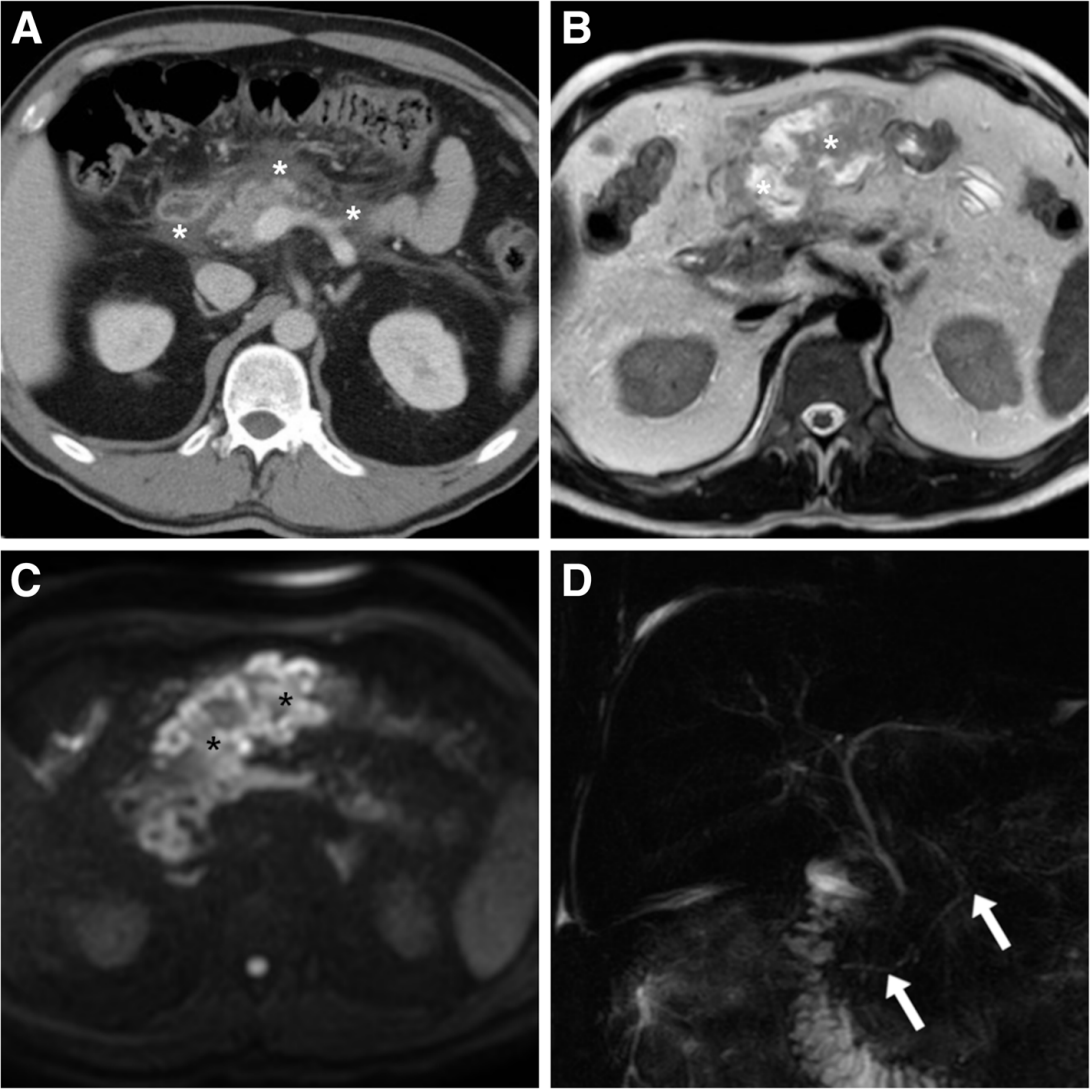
In a 44-year-old male with clinical and laboratory features consistent with mild AP, early contrast-enhanced MDCT (a) showed preserved pancreatic enhancement and mild peripancreatic oedema and fluid (*). A week later, MRI including T2-weighted (b) and diffusion-weighted (DW, c) showed development of a vast heterogeneous region of peripancreatic fat necrosis (*). Additionally, MRCP (d) showed pancreas divisum (MPD indicated by arrows draining into the minor papilla) as the underlying cause of AP. Afterwards, the patient had MPD stenting (not shown) through the Santorini duct to prevent recurrence of AP
